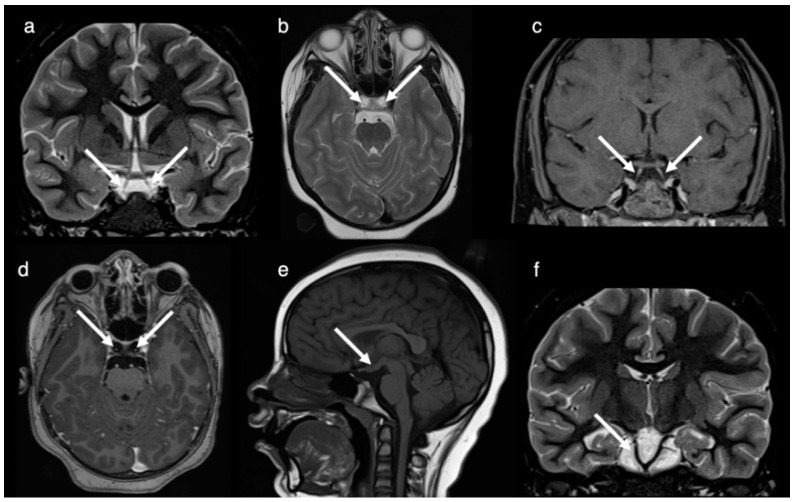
Brain MRI images obtained at 8 years of age. Coronal T2-weighted short-tau inversion recovery (STIR; (a)), axial T2-weighted (b), coronal fat saturated T1+C-weighted (c) and axial T1+C-weighted (d) MR-images demonstrate the complete duplication of the pituitary gland including the pituitary stalk (arrows in (a–d)). Sagittal T1-weighted MR-image (e) documents the tuberomammillary fusion (arrow). Coronal T2-weighted STIR image (f) shows the duplication/low bifurcation of the basilar artery (arrow) at the midpons level with the distal basilar artery supplying the superior cerebellar and posterior cerebral arteries.
